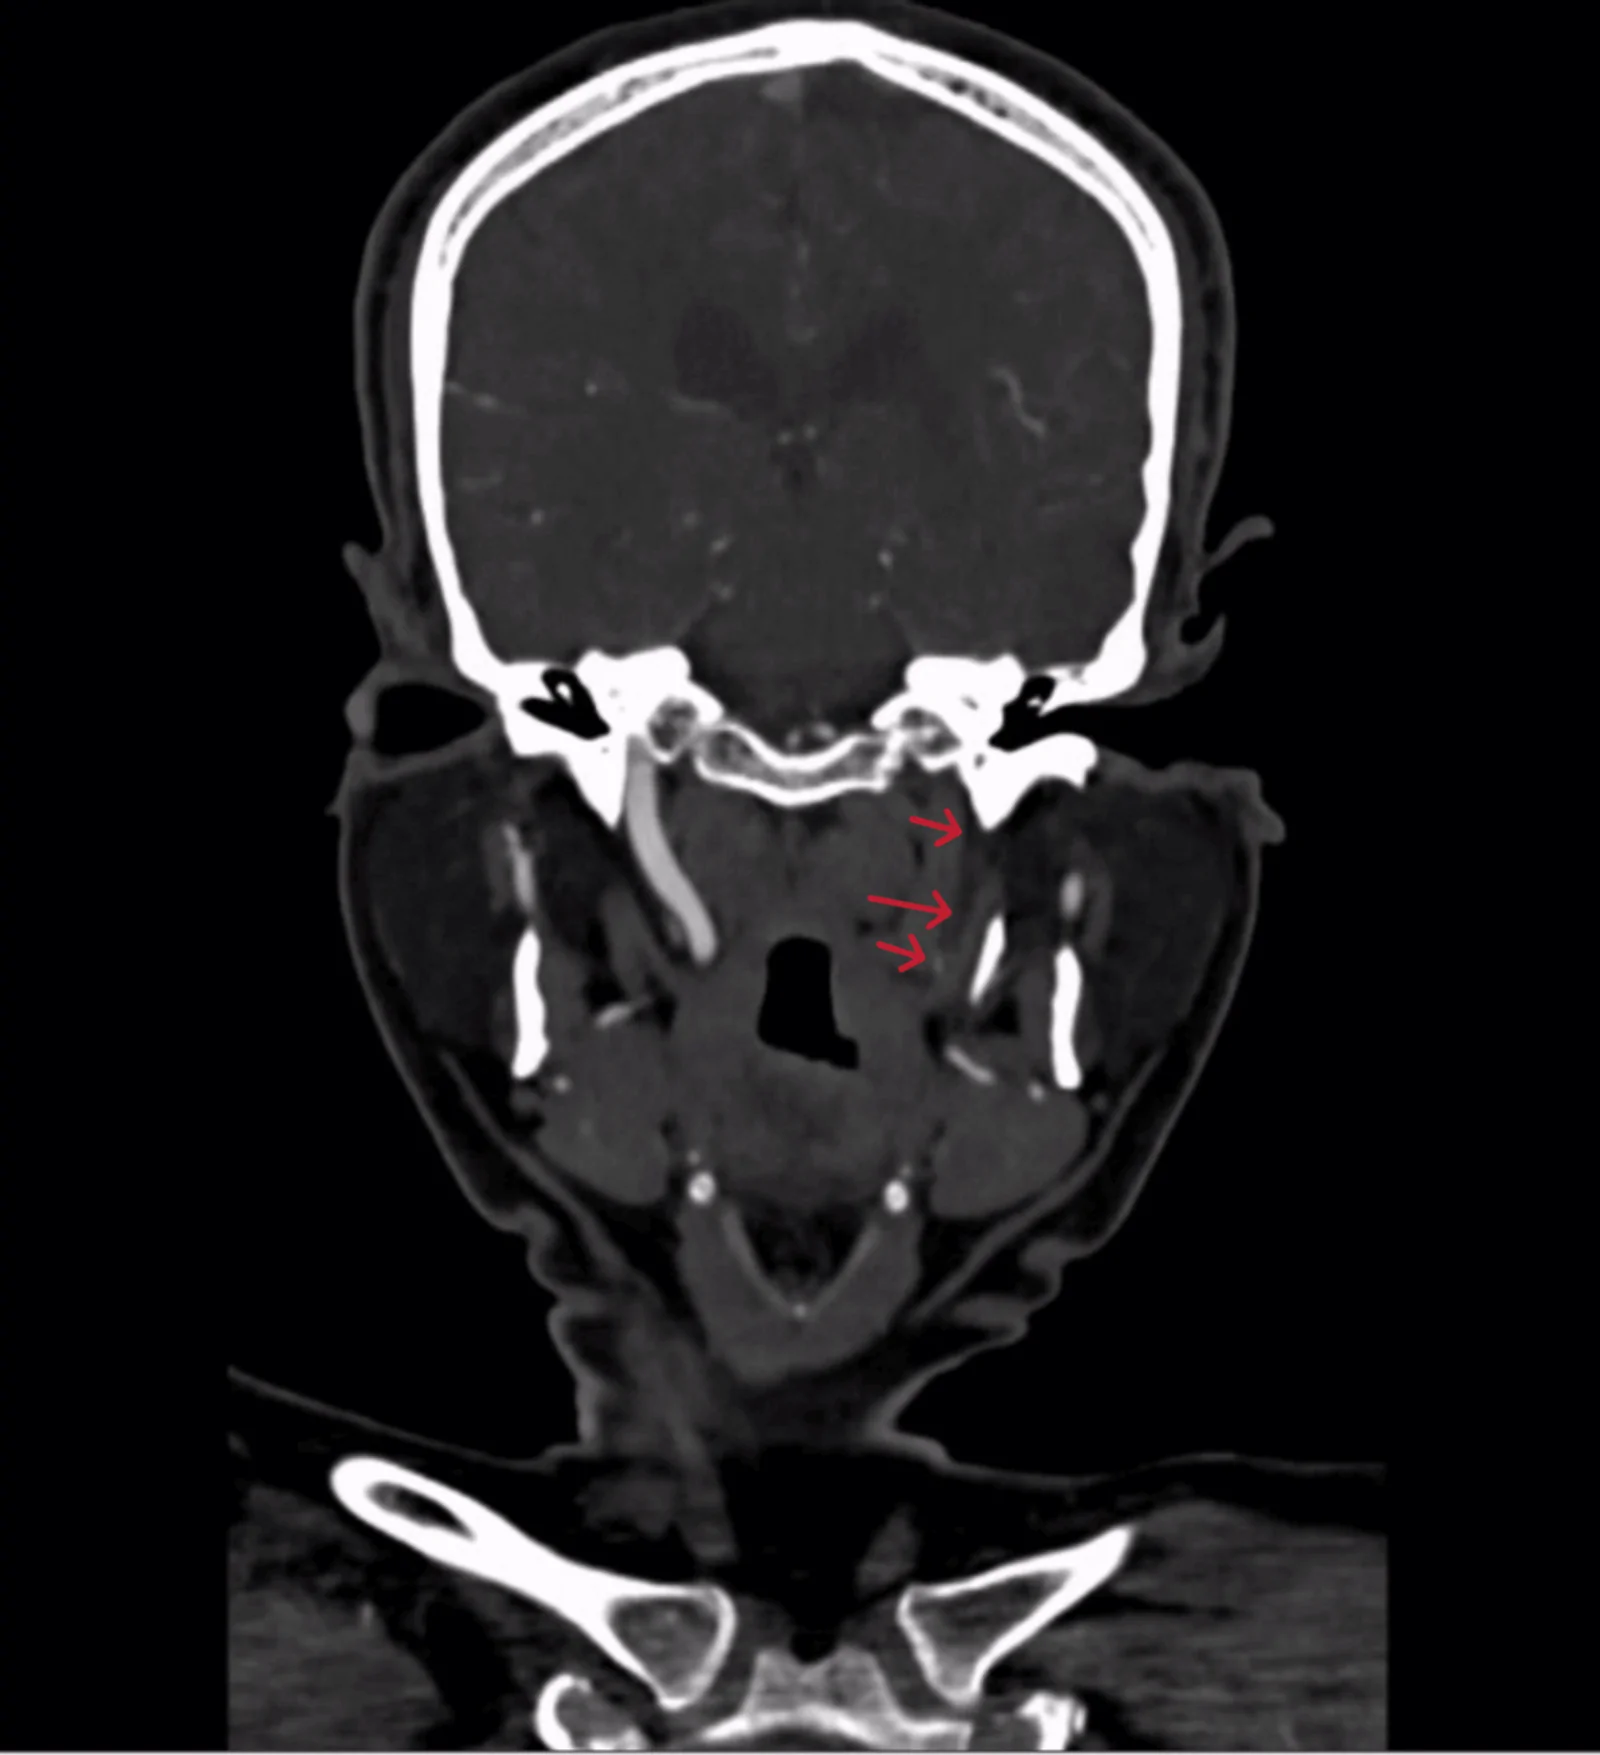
CT angiography (CTA) of the head and neck demonstrating filling defect of the left internal carotid artery (as indicated by red arrows).
